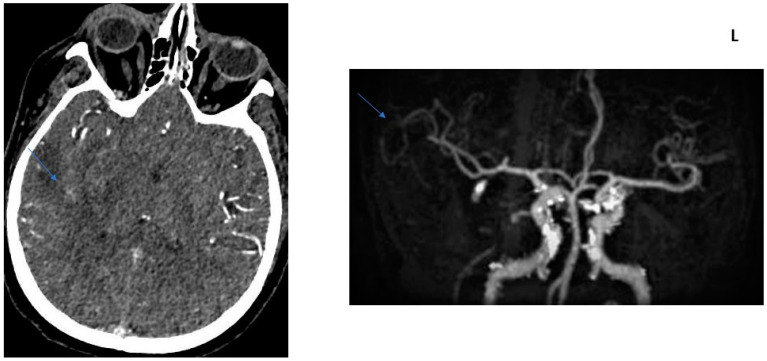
COLLATERAL SCORE: 1–68-year-old female patients. Right MCA infarction. NIHH Score: 14. CT angiography was performed 4.5 h after the acquisition of clinical findings. Left image, “Subtracted MIP image obtained from arterial phase images”. Right image, “Raw image in the axial plane obtained in the arterial phase after contrast injection”. Arrows represent collateral score area.
